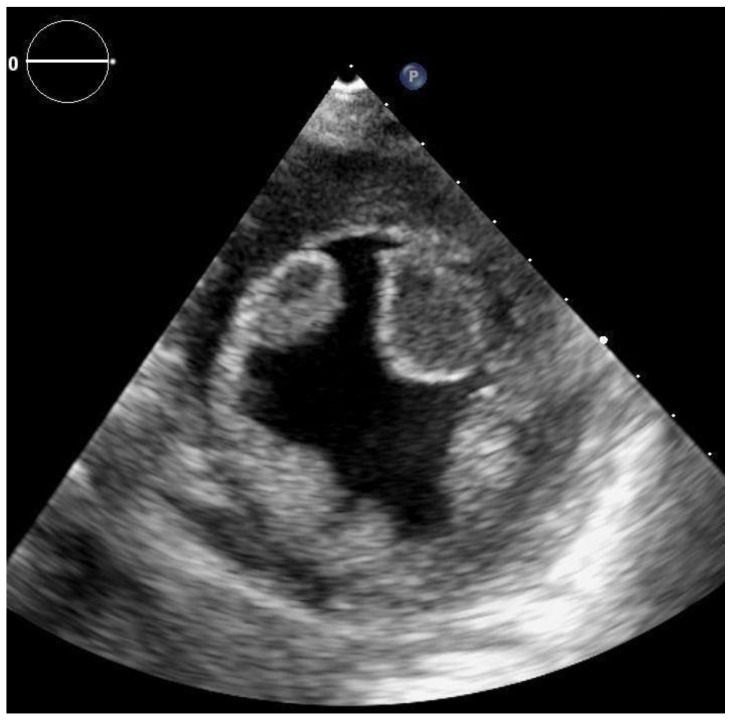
2D transthoracic echocardiography, parasternal short axis view at the level of papillary muscles—visualization of apical-septal (AS) and -lateral (AL) thrombi in the anterior part of the left ventricle.
